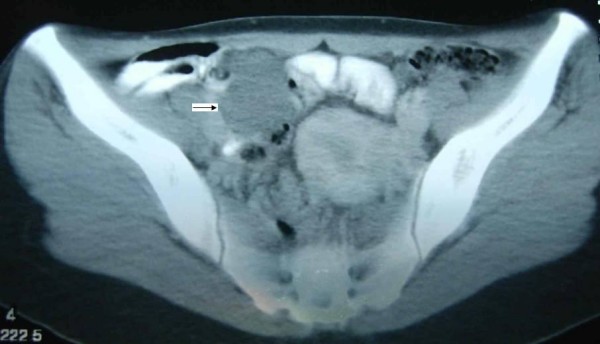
Oral and intravenous contrast enhanced computed tomography scan showing the mesenteric mass of the ileal small bowel segment (arrow).
